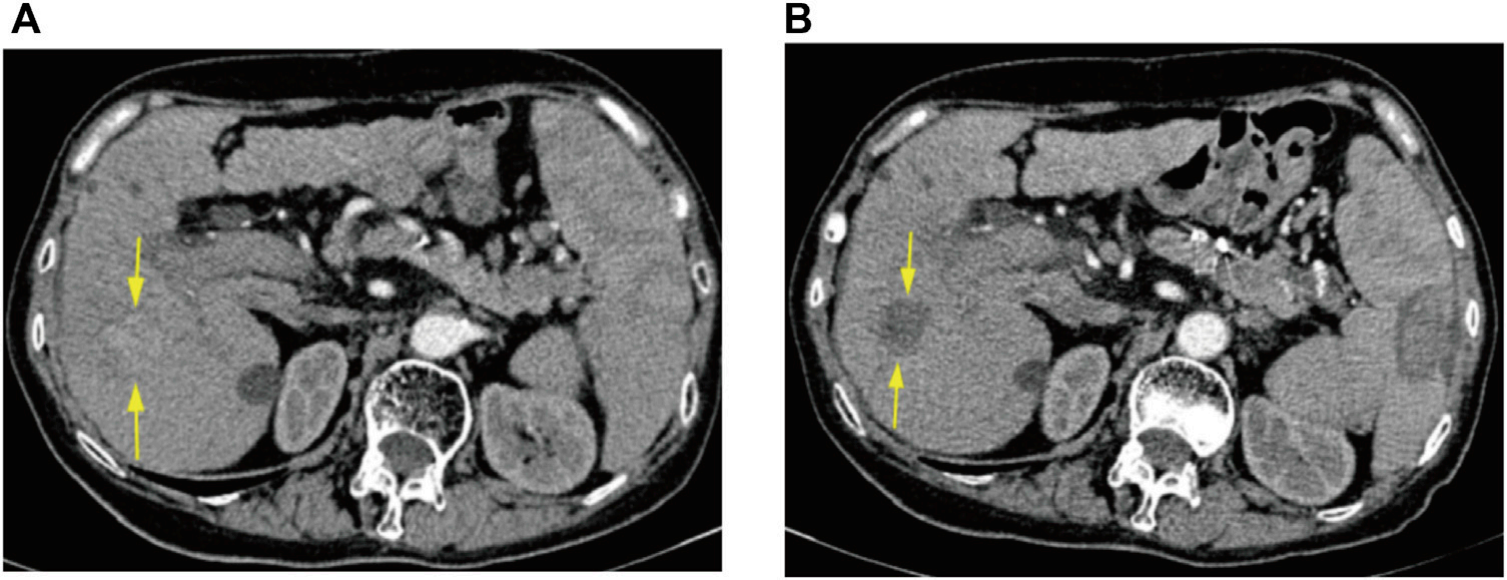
CT arterial phase images of a 75-year-old female patient before DEB-TACE and at 6 weeks postoperative re-examination. (A) Preoperative enhanced CT representing HCC in the S5 segment of the liver. (B) Postoperative enhanced CT demonstrating no enhancement of surviving tumor in the area of the original lesion, with efficacy assessed as CR.
